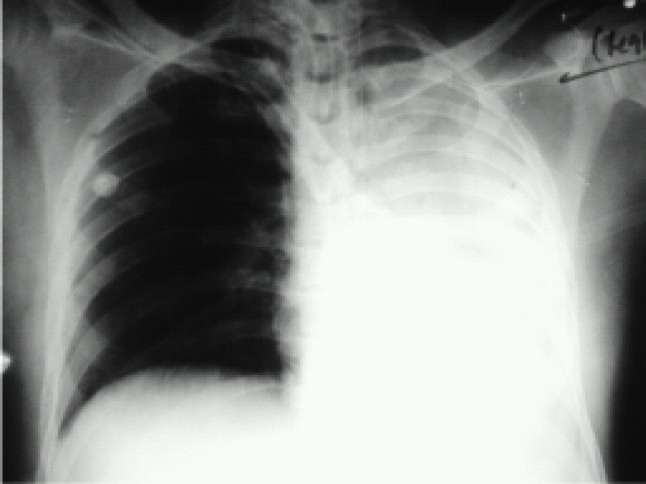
Chest X-ray on 6th day of ventilation showing VAP
